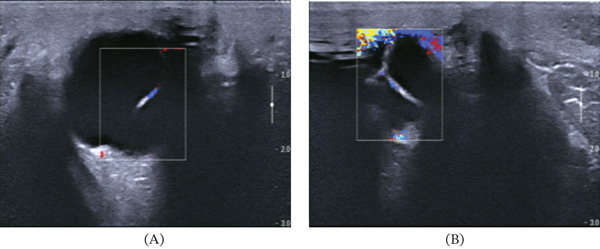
Color Doppler ultrasound images. (A) 2D ultrasound and color Doppler flow imaging (CDFI) of the right eye shows a thin, medium echogenic band connected from the posterior lens to the optic disc, in an “I” shape. (B) 2D ultrasound and CDFI of the left eye show a similar echogenic band. Short linear blood flow signals are visible within the band on CDFI.
